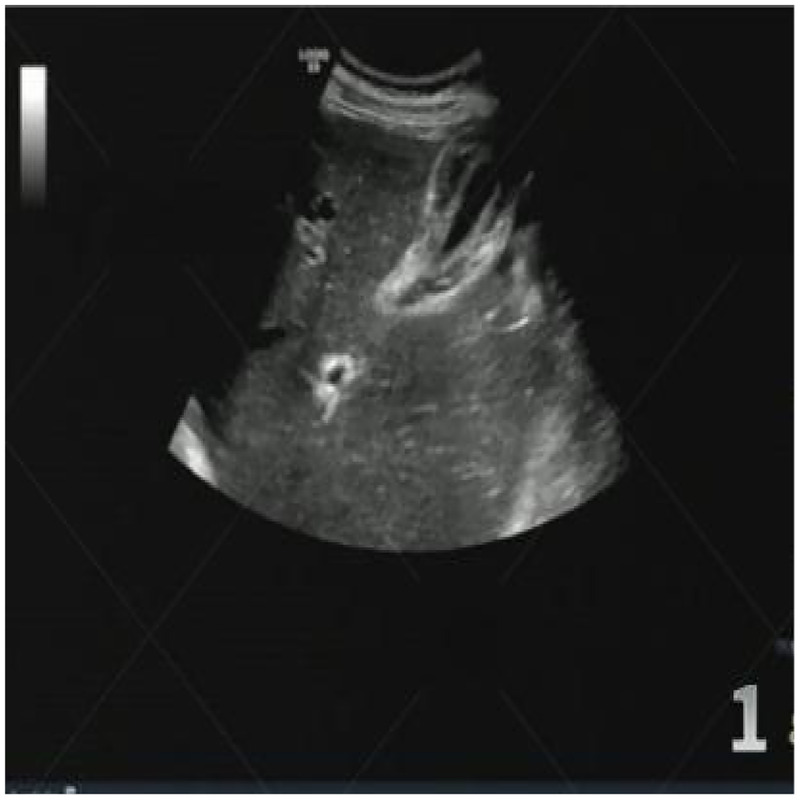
Ultrasound revealed edema of the gallbladder wall.
